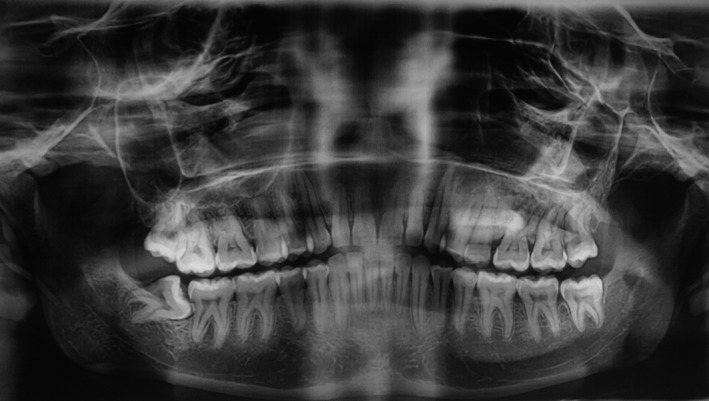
Orthopantomogram showing mixed radiolucent and radiopaque mass with diffuse border in the left maxilla.
